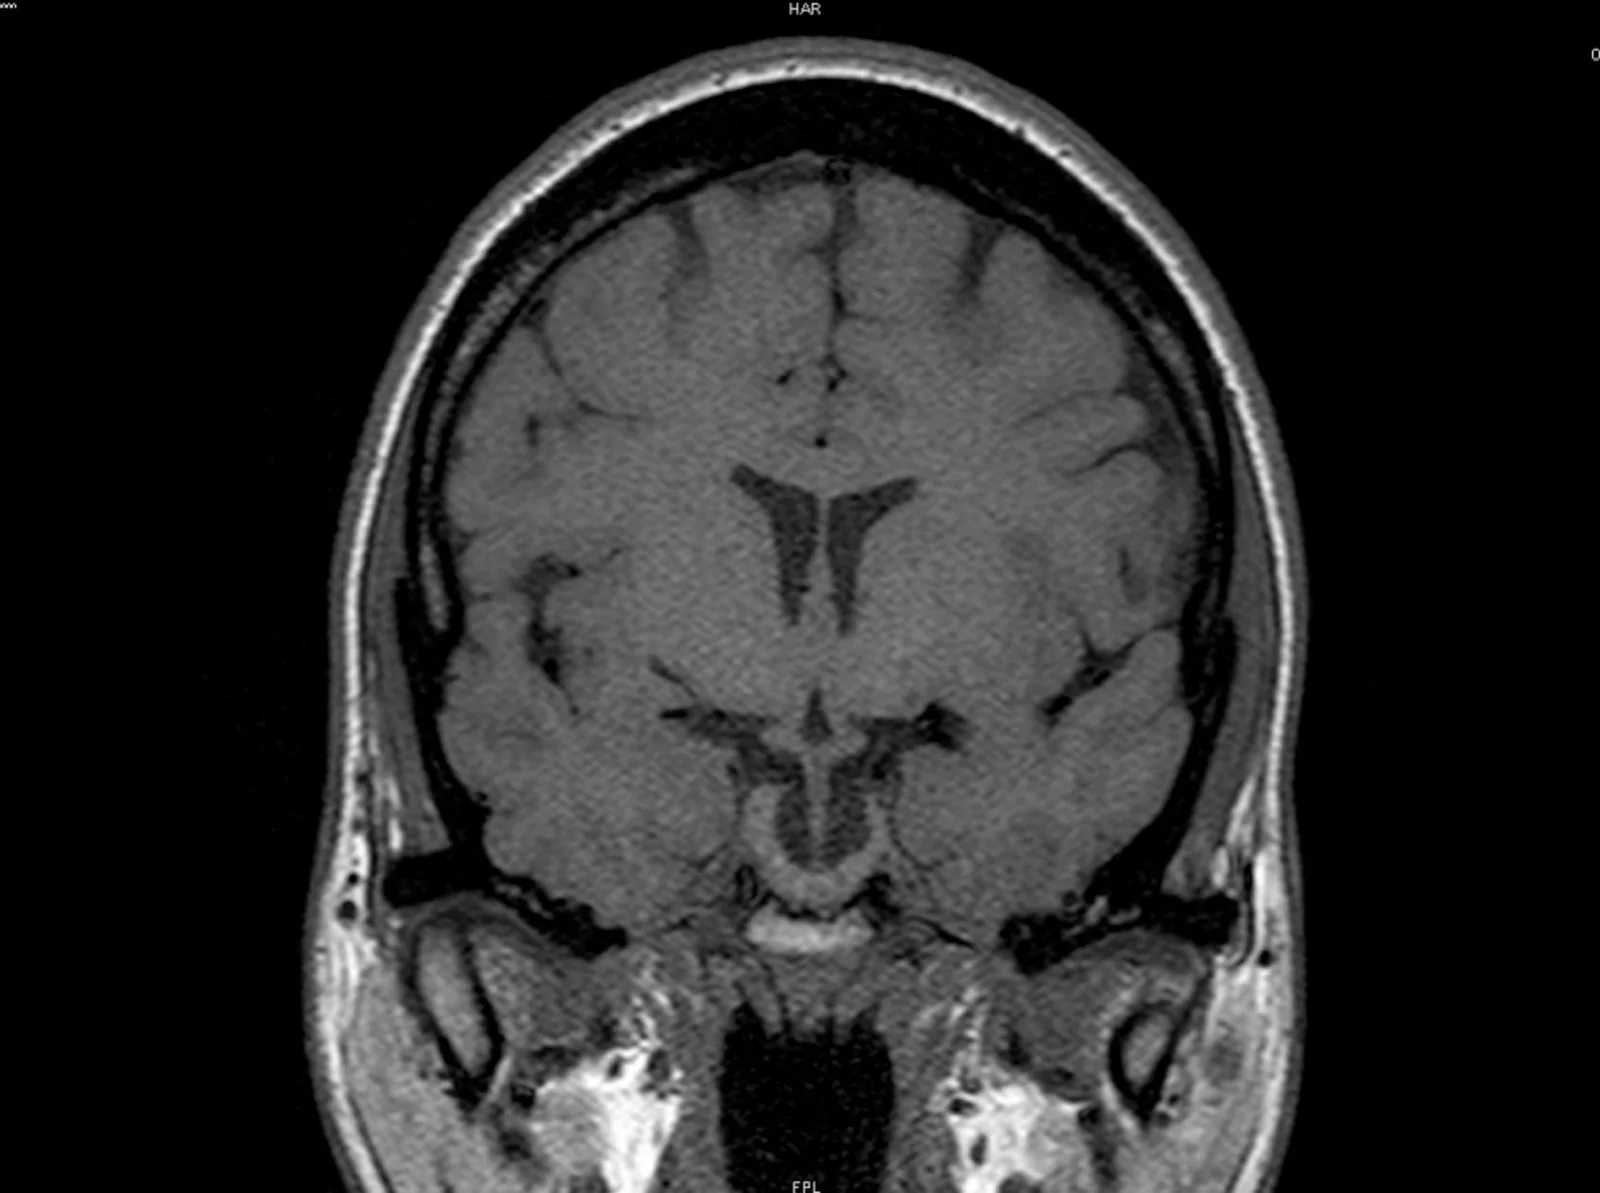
Pituitary MRI with no pituitary lesions identified.
